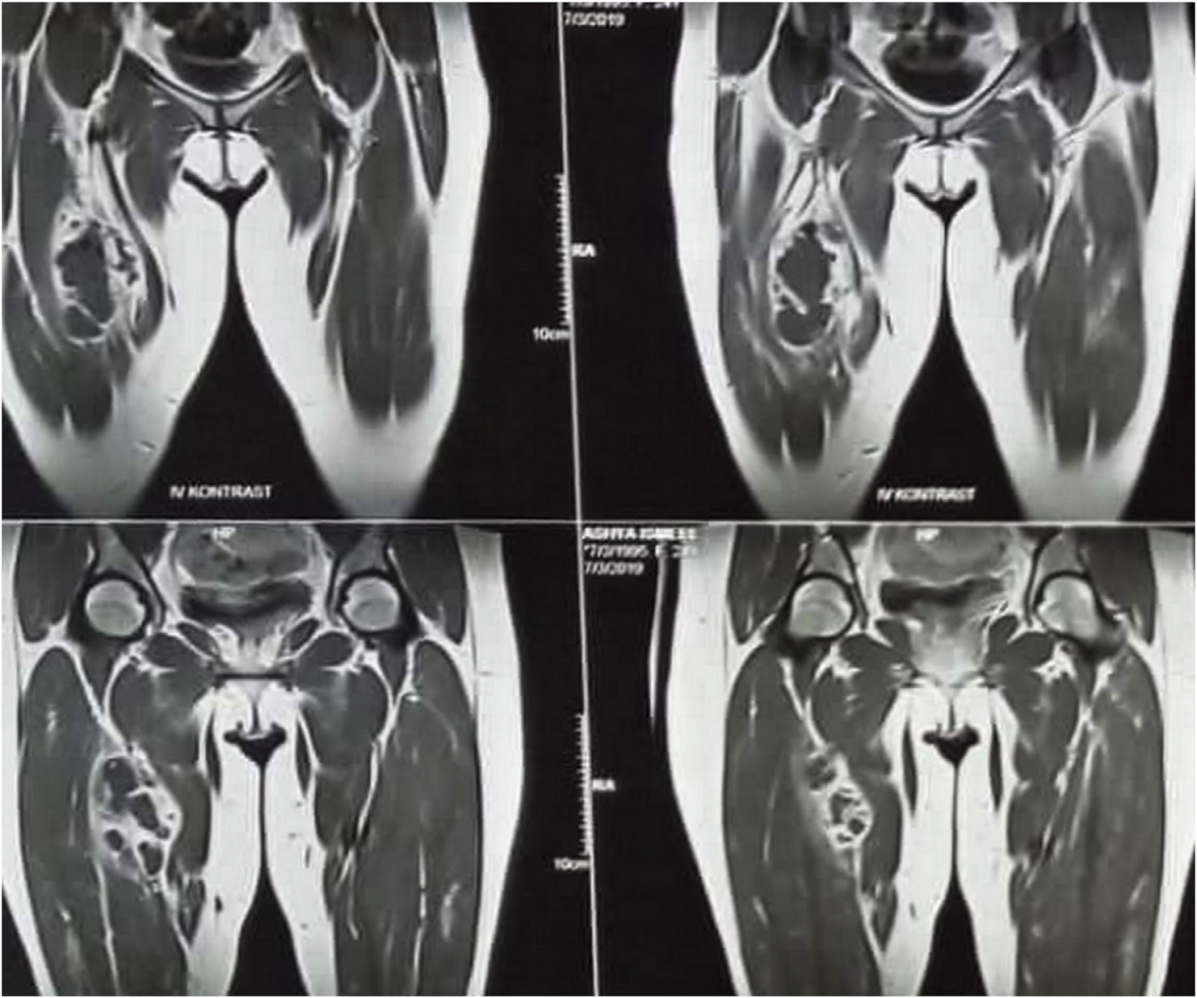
T1-weighted MRI study of the thigh showing a multi-loculated cystic lesion in the right thigh with multiple internal septations.
